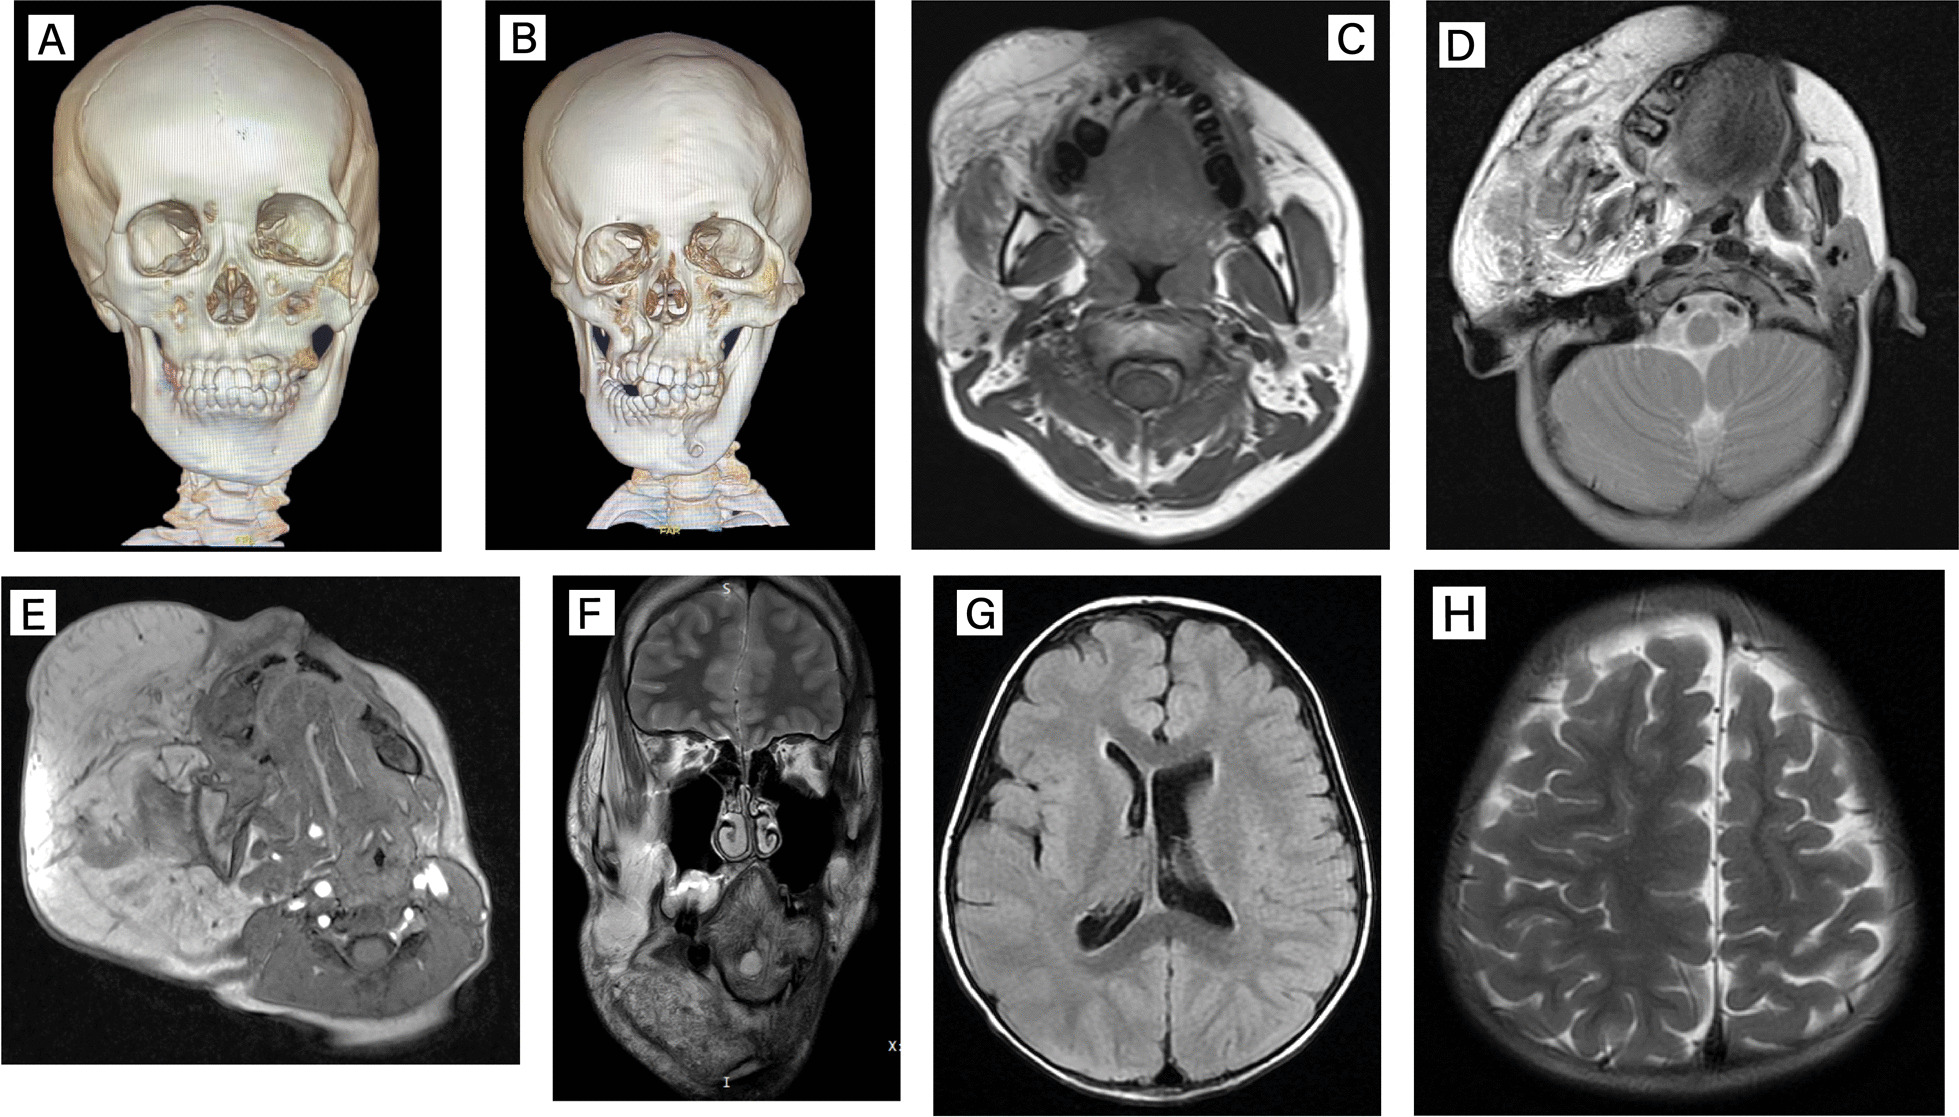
Maxillofacial imaging findings. A Stereoscopic image of the skeleton of Patient #6 provided by CT 3D reconstruction. Most patients had potential skeletal overgrowth, including zygomatic, maxillary, and mandibular bones. B The 3D reconstructed image of Patient #11. Excessively large lipomatosis can compress the surrounding structures, causing the bones to develop malformations while overgrowing and interfering with the normal growth and alignment of the teeth. C–E Magnetic resonance (MR) images of Patients #8, #7, and #11. Infiltrative growth of lipomatosis can have a variable impact. As shown in C, enlarged occlusal muscles and parotid glands are very prominent features, and mild infiltration of adipose tissue does not affect the normal anatomy of the maxillofacial region. As shown in (D, E), more severe diffuse infiltration of adipose tissue makes facial structures confusing and difficult to distinguish and can be detrimental to the patient’s function. F T2 coronal image of Patient #5. The aberrant signal and structure near his right mandible seemed to indicate that this was a lymphatic malformation. G, H Cranial MRI of Patient #1 revealed asymmetric brain development and an enlarged left ventricle
